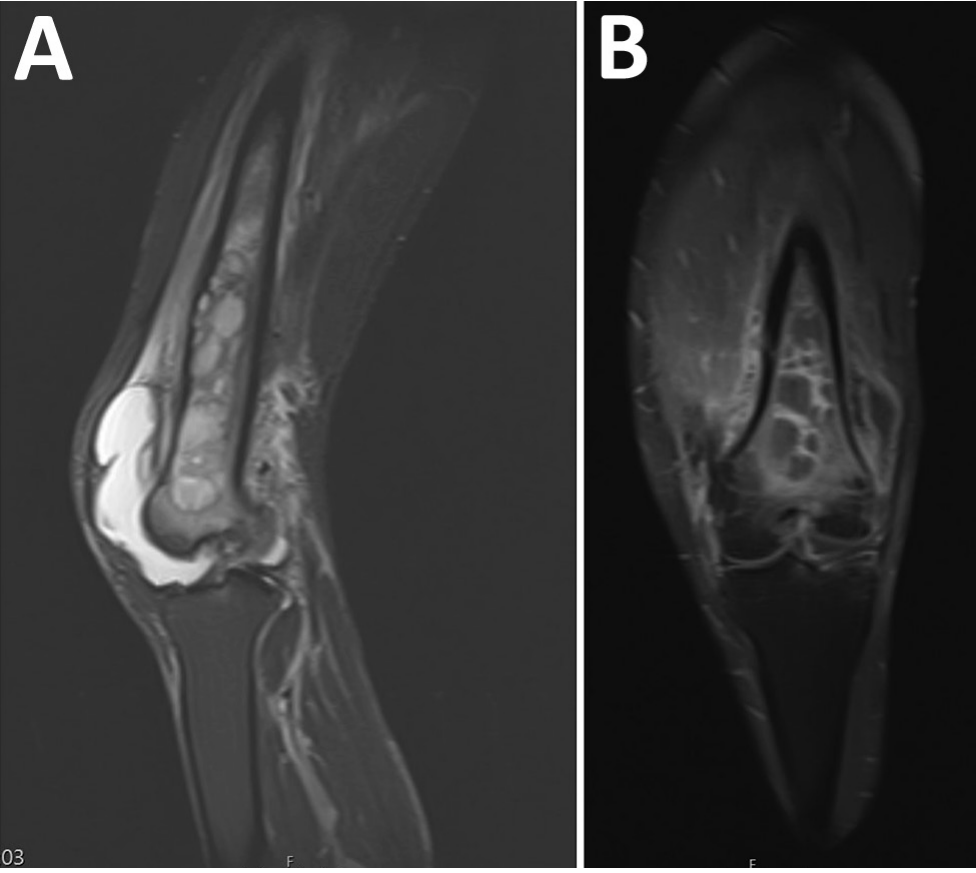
Melioidosis manifesting as chronic femoral osteomyelitis of the left leg in patient from Ghana. A) Magnetic resonance imaging showing extensive femoral osteomyelitis. FFB) Magnetic resonance imaging showing extensive osteomyelitis.
